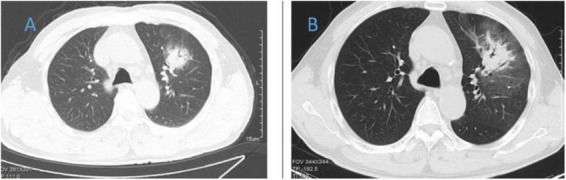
Chest CT of patient 6. (A) Before treatment on 2021.10.19. (B) The follow-up imaging 1 week after treatment showed progression compared to the pre-treatment scans.
